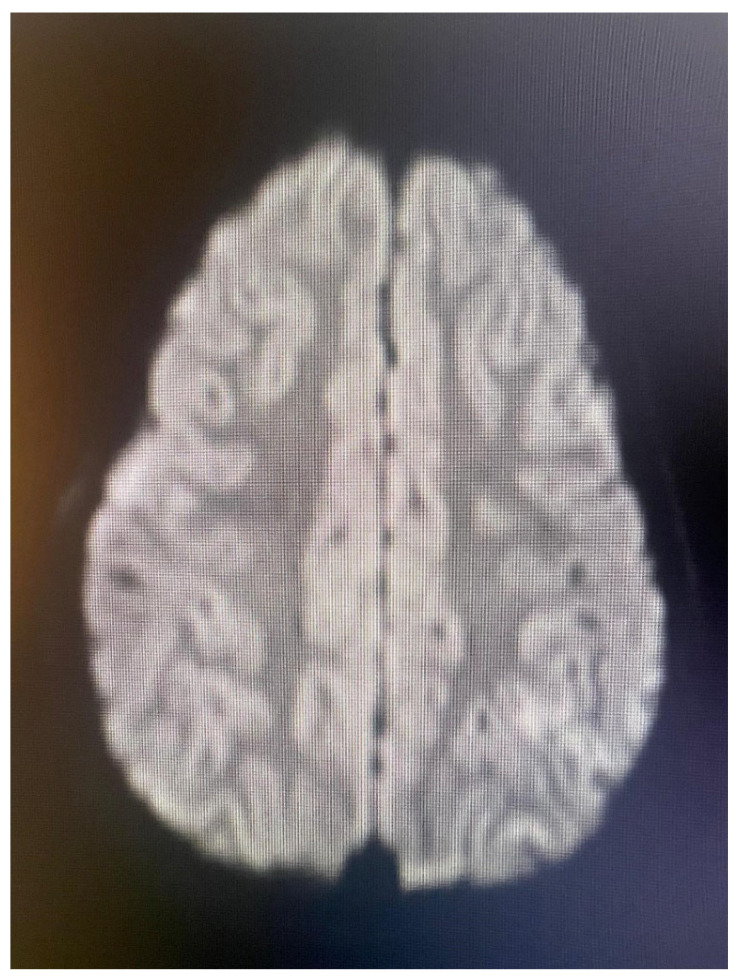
A 6-year-old boy with FIRES (febrile illness refractory epilepsy syndrome), precedent infections include Rhinovirus and Enterovirus. MRI showed foci of gyriform cortical diffusion restriction in the left. parietal lobe and left temporal lobe, with associated relative hypo-enhancement of these cortices, and to a lesser extent at the left hippocampus and right lateral frontoparietal cortex. There is no associated hyper-enhancement or increased FLAIR signal. The MRI findings suggest a diffuse process, but most prominent in the same areas of the EEG.
